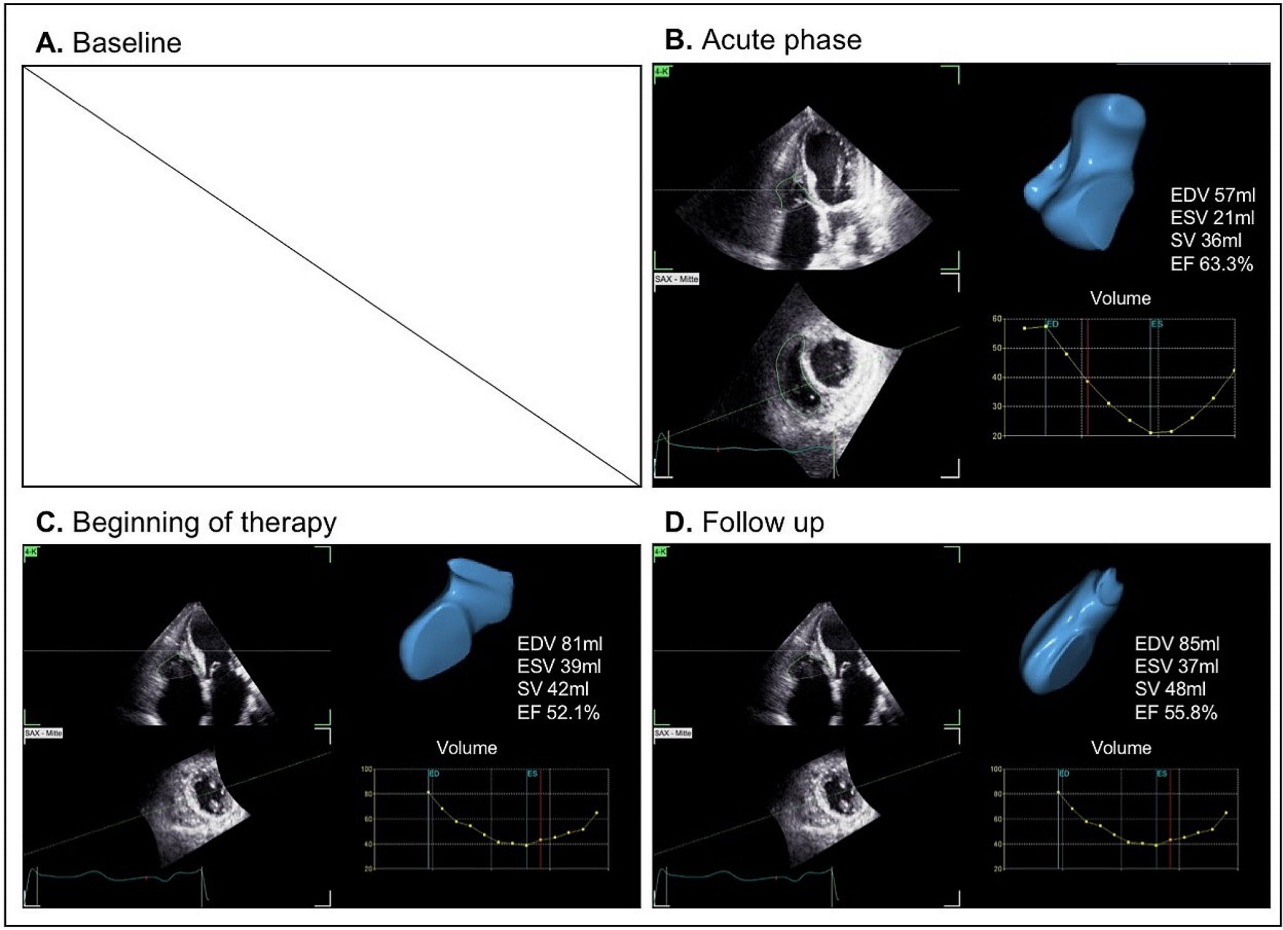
Right ventricular (RV) volume excluding thrombus formation. No 3 data were acquired at baseline (A), RV volume analysis at acute stage of inflammation (B), at early prednisolone therapy (C) as well as two weeks under ongoing immunosuppressive therapy (D). At baseline no 3D data acquisition was performed at routine echocardiography (A). In the acute phase RV end-diastolic volume (RV-EDV) was 57 ml and RV end-systolic volume (RV-ESV) was 21 ml (B). At start of prednisolone therapy RV-EDV was 81 ml and RV-ESV was 39 ml (C). During the later follow up RV-EDV was 85 ml and RV-ESV was 37 ml, respectively (D). The calculated RV total stroke volumes excluding the RV thrombus formation are 36 ml, 42 ml, and 48 ml, respectively
